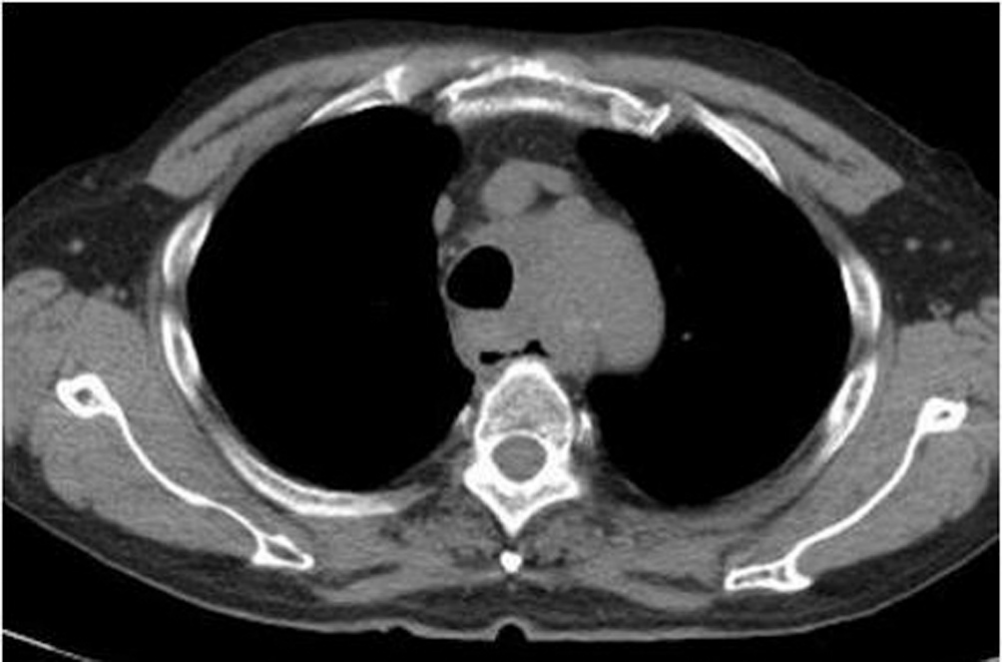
Mediastinal lymph node decreased in size on plain CT 1 week following completion of radiotherapy at the level of the aortic arch.
